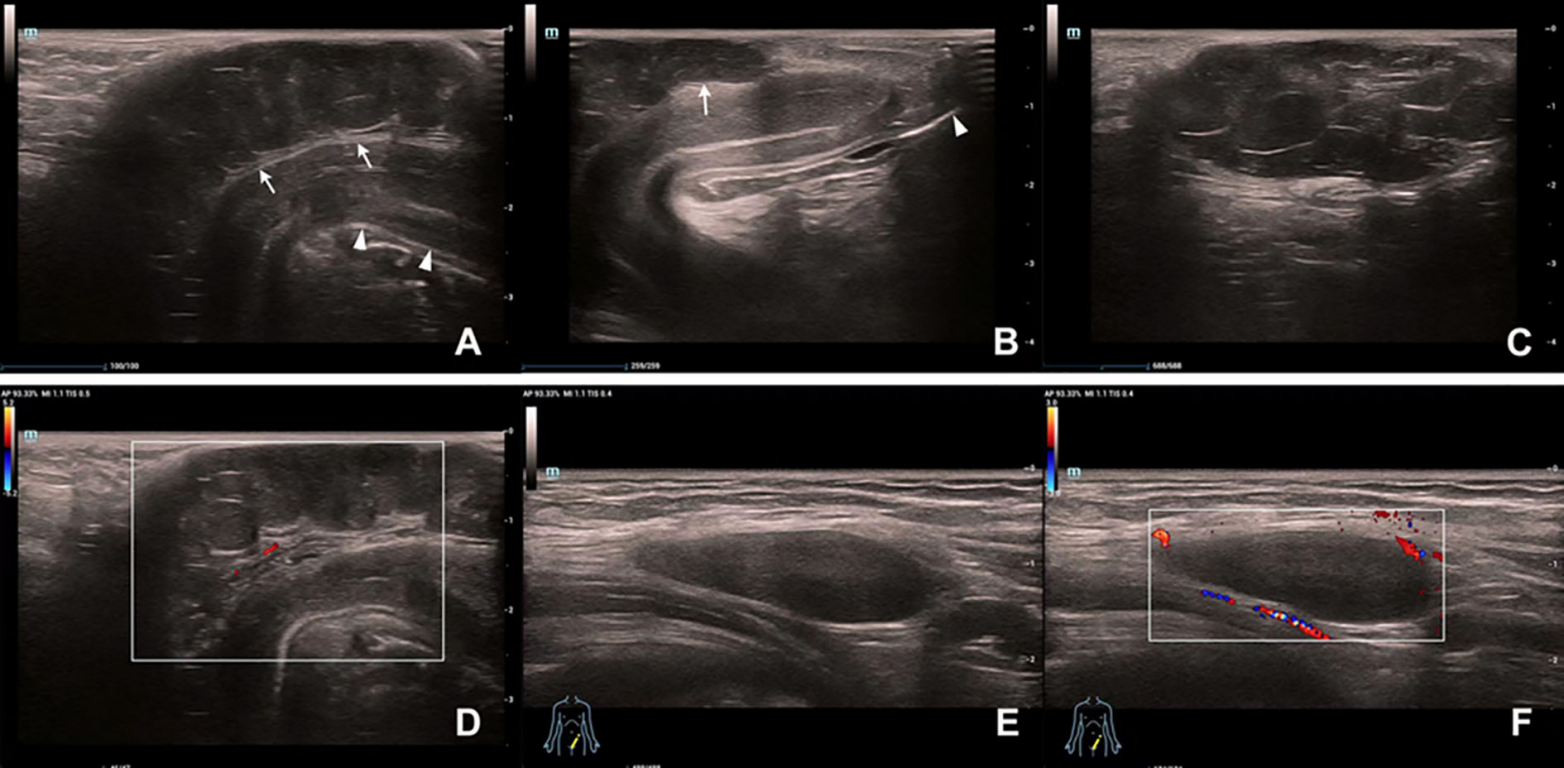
Ultrasound findings of the masses on the penis and in the left groin area. (A) A hypoechoic mass (arrow) with a hyperechoic capsule on the dorsal side of the penile shaft (arrowhead). (B) The distal part of the mass (arrow) does not involve the glans (arrowhead). (C) Posterior acoustic enhancement and multiple linear hyperechoic separations can be seen inside the penile mass. (D) Vascularity of the penile mass was not abundant on color Doppler flow evaluation. (E) A homogeneous hypoechoic mass in the groin area. (F) The inguinal mass showed hypovascularity on Color Doppler ultrasonography.
